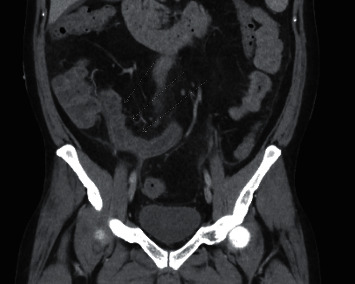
Coronal CT image with IV contrast demonstrating inflammatory changes of the terminal ileum which has a thickened wall.
